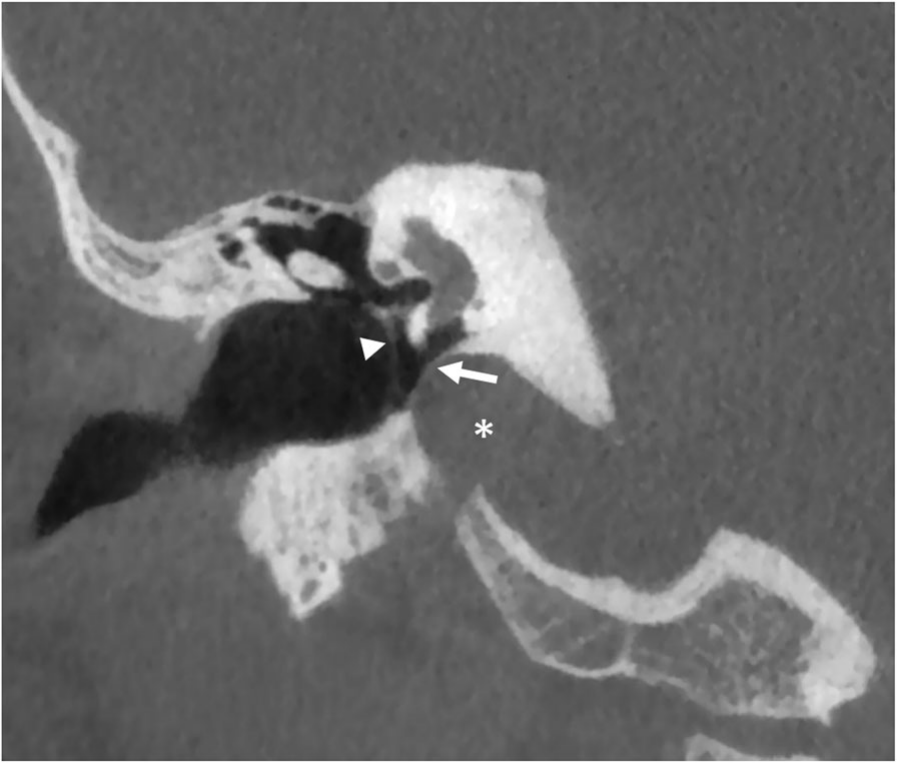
Jugular bulb protrusion*.* Coronal CBCT scan shows prominence of the right jugular bulb (asterisk) into the hypotympanic cavity; the bony plate covering the vein is thinned (arrow). Tympanic membrane (arrowhead)
